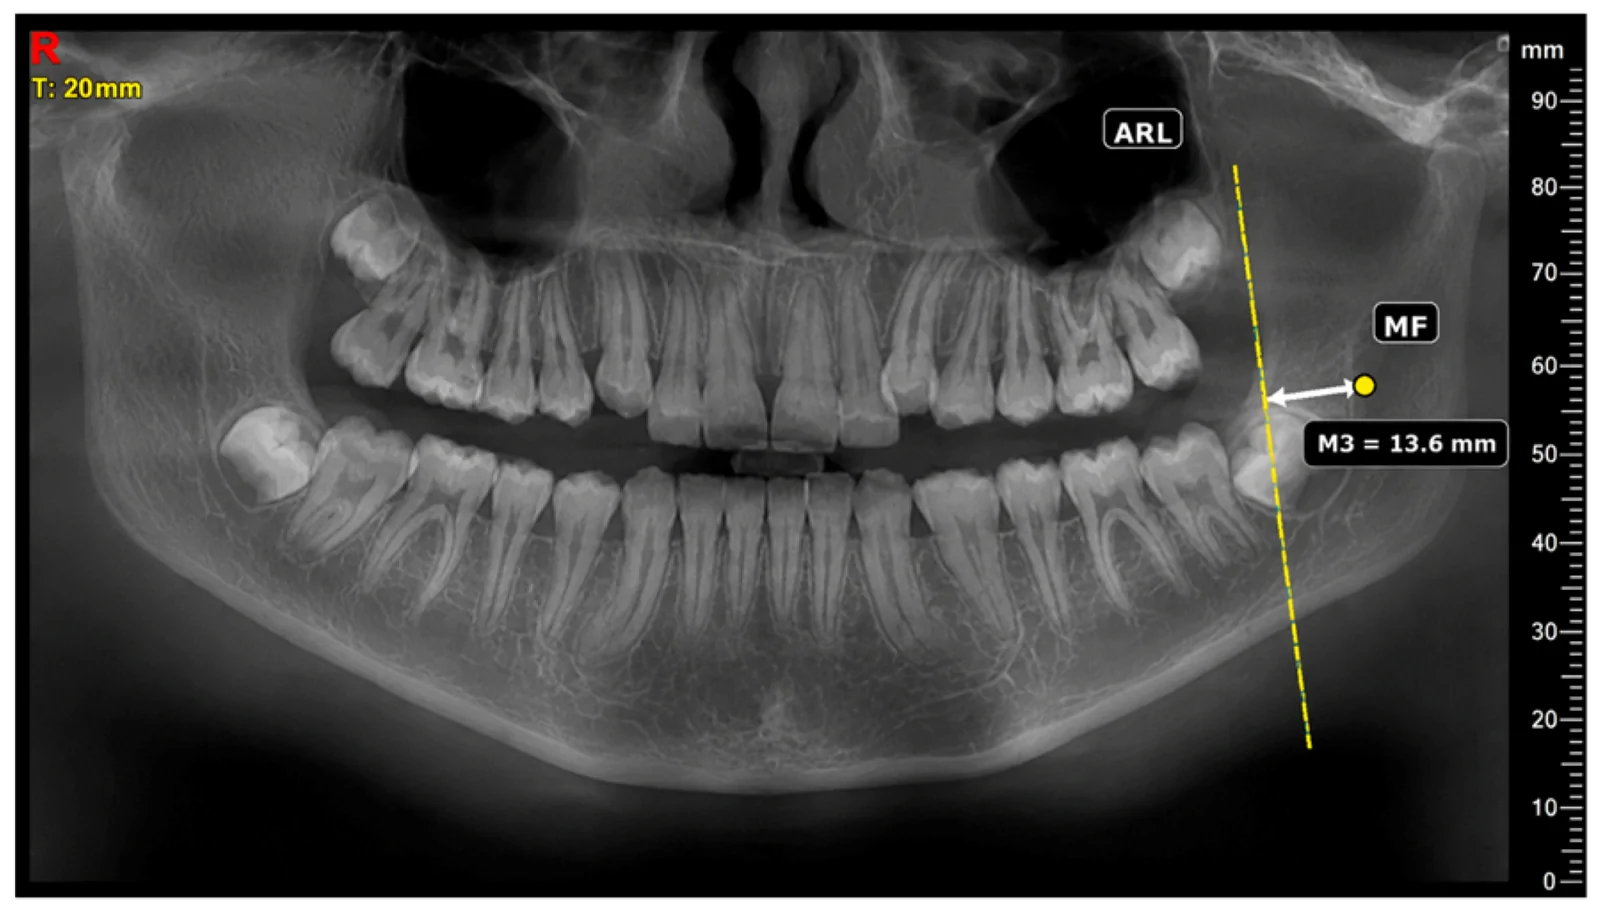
CBCT-derived panoramic reconstruction showing the M3 measurement (mm), defined as the shortest perpendicular distance from the fixed superior mandibular foramen reference point (MF) to the anterior ramal reference line (ARL).
